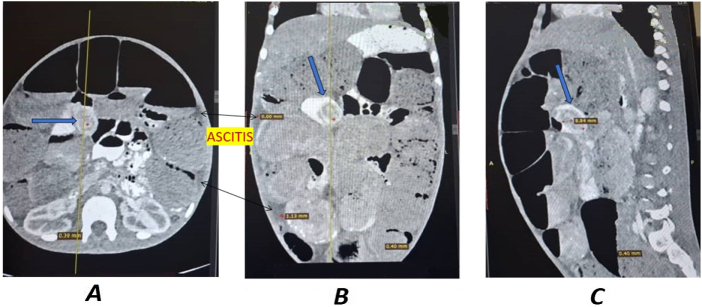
Hepatic flexure colonic mass, large bowel loops edematous and dilated. (A) CT-scan axial view. (B) CT-scan coronal view. (C) CT-scan sagittal view.
